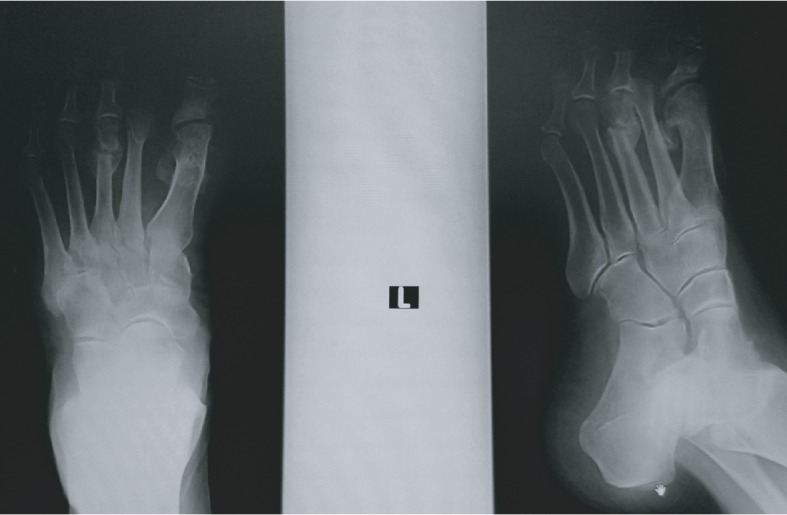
X-ray of the patient's left foot three months after the operation.
